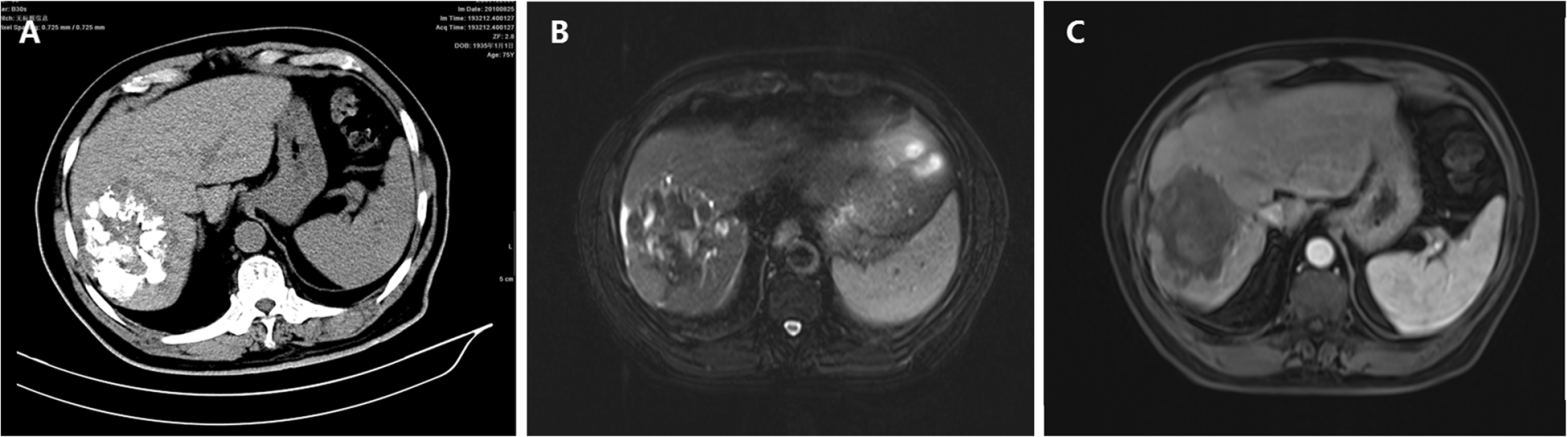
Incomplete ablation of HCC. A: CT image before RFA; B and C: MRI image after RFA.
